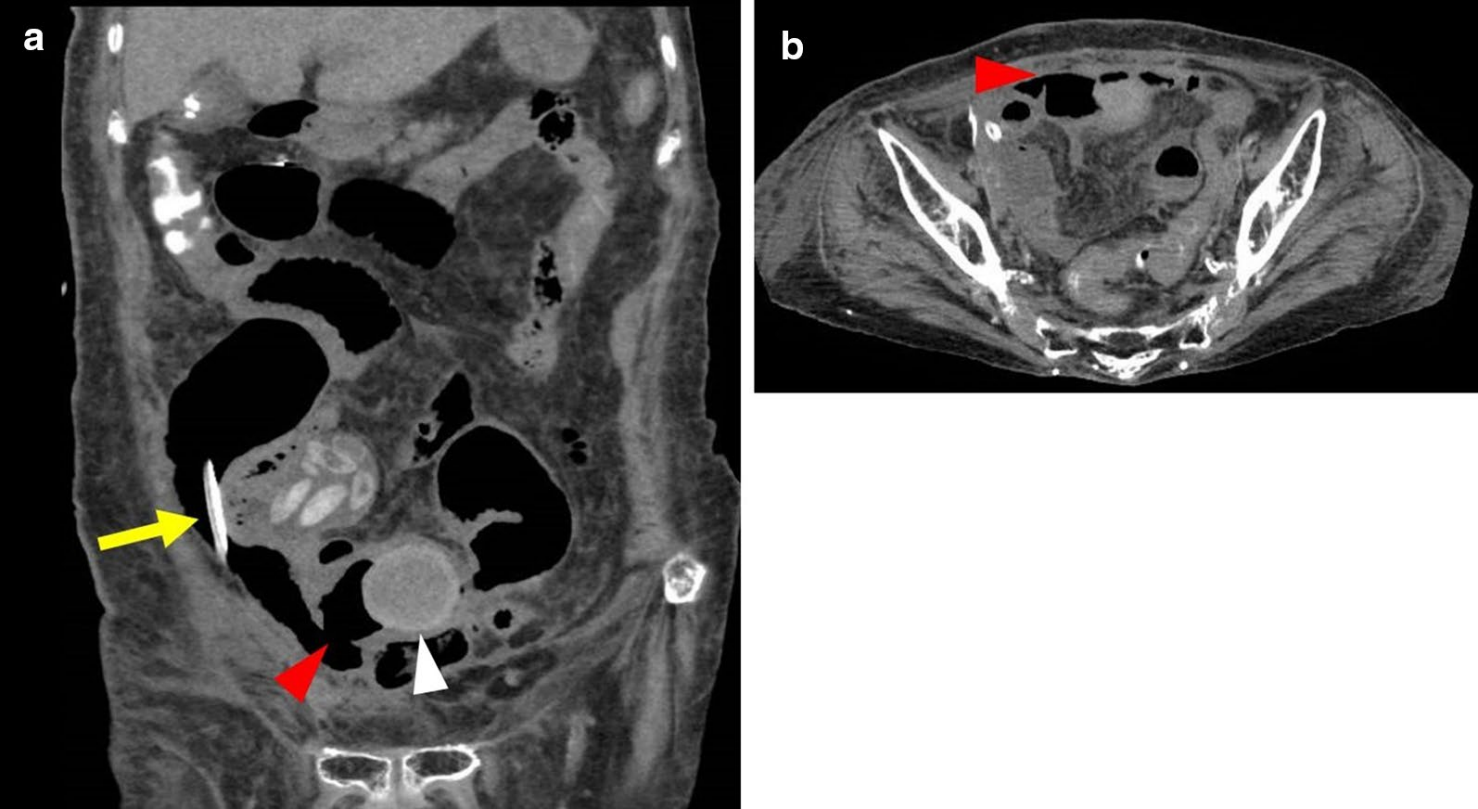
Follow-up abdominal plain CT performed 10 days after onset. a Coronal and b transaxial images reveal that the abdominal free space, including gas and the drainage tube (yellow arrow), communicates with the intraluminal space of the small intestine, which contains a slightly high-density spherical structure (white arrowhead) in the vicinity, via the perforation site (red arrowheads)
